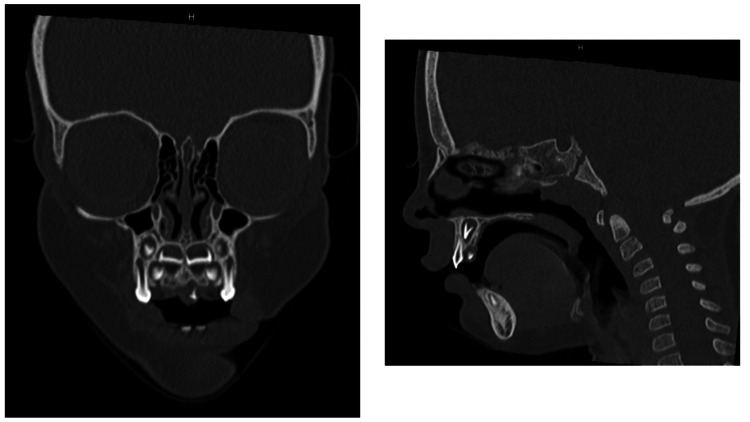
CT scan showing a radiopaque fusiform mass underlying the oral lesion with intact palatal bone.
